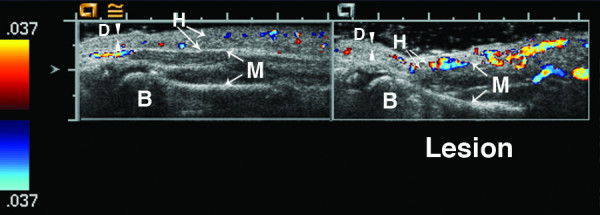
6 year old girl with a four year history of linear scleroderma of her left arm and hand. Her lesion has extended to her upper back, and she has new lesions on her face and chest. The imaged area is the volar surface of hand above 5^th ^metacarpal bone, which shows chronic atrophy including shortening of this portion of her hand, but no signs of activity. The patient complained of pain in this area. There is abundant color Doppler signal in both the normal and lesion area, but more vascularity is seen in the lesion hypodermis and muscle layers. The lesion hypodermis shows a large area of contiguous color Doppler signal that encompasses at least 20% of the surface area of the imaged hypodermis (hypodermal vascularity = 3). There are 2 large color Doppler signals in the lesion muscle and none in the normal muscle, giving a deep tissue vascularity score of 2. B = bone, D = dermis, H = hypodermis, and M = muscle. Arrowheads indicate boundaries of tissue layers.
